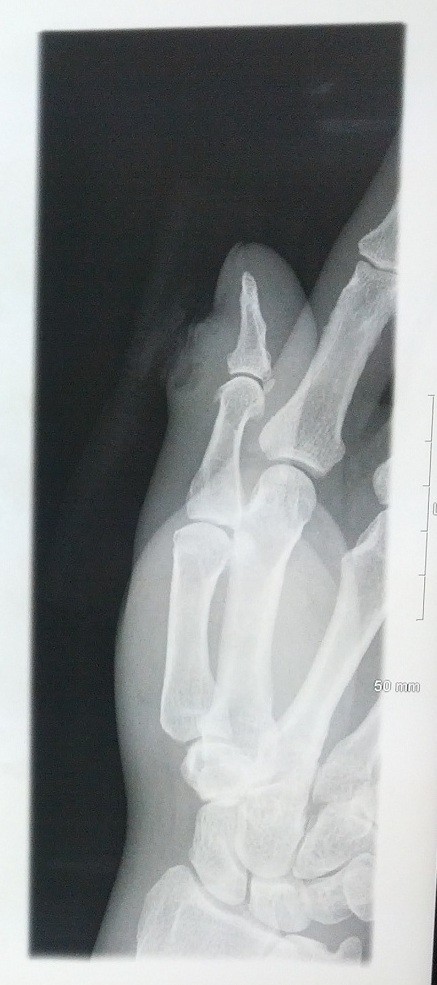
Right thumb radiographs taken on admission to the hospital. Plain films show cellulitis and edema of the skin overlying the interphalangeal joint of the first digit. There is no evidence of fracture, dislocation, or osteomyelitis.
